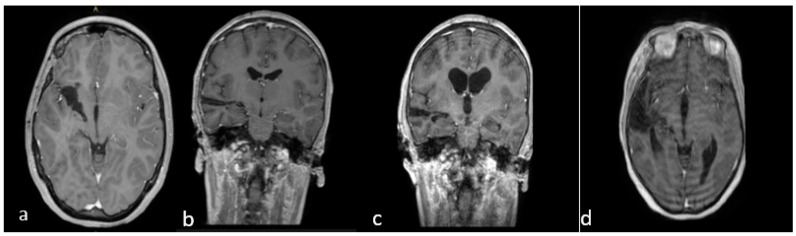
(a–d) Brain MRI, T1-weighted with gadolinium. Last follow-up of Case A (a,b) and Case B (c,d). (a,b) Brain MRI, T1-weighted with gadolinium, in the axial and coronal planes of Case A, showing good control of the disease (2021). (b–d): MRI, T1-weighted with gadolinium, in the coronal and axial planes of Case B, showing no evidence of relapse five years post-op.
